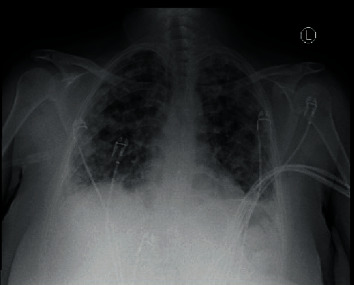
Chest X-ray showing worsening bilateral nodular opacities on hospital day 1.
